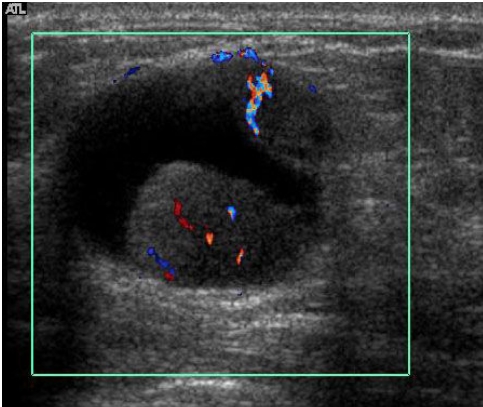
Transverse color Doppler US image shows vascular flow within the solid components.
